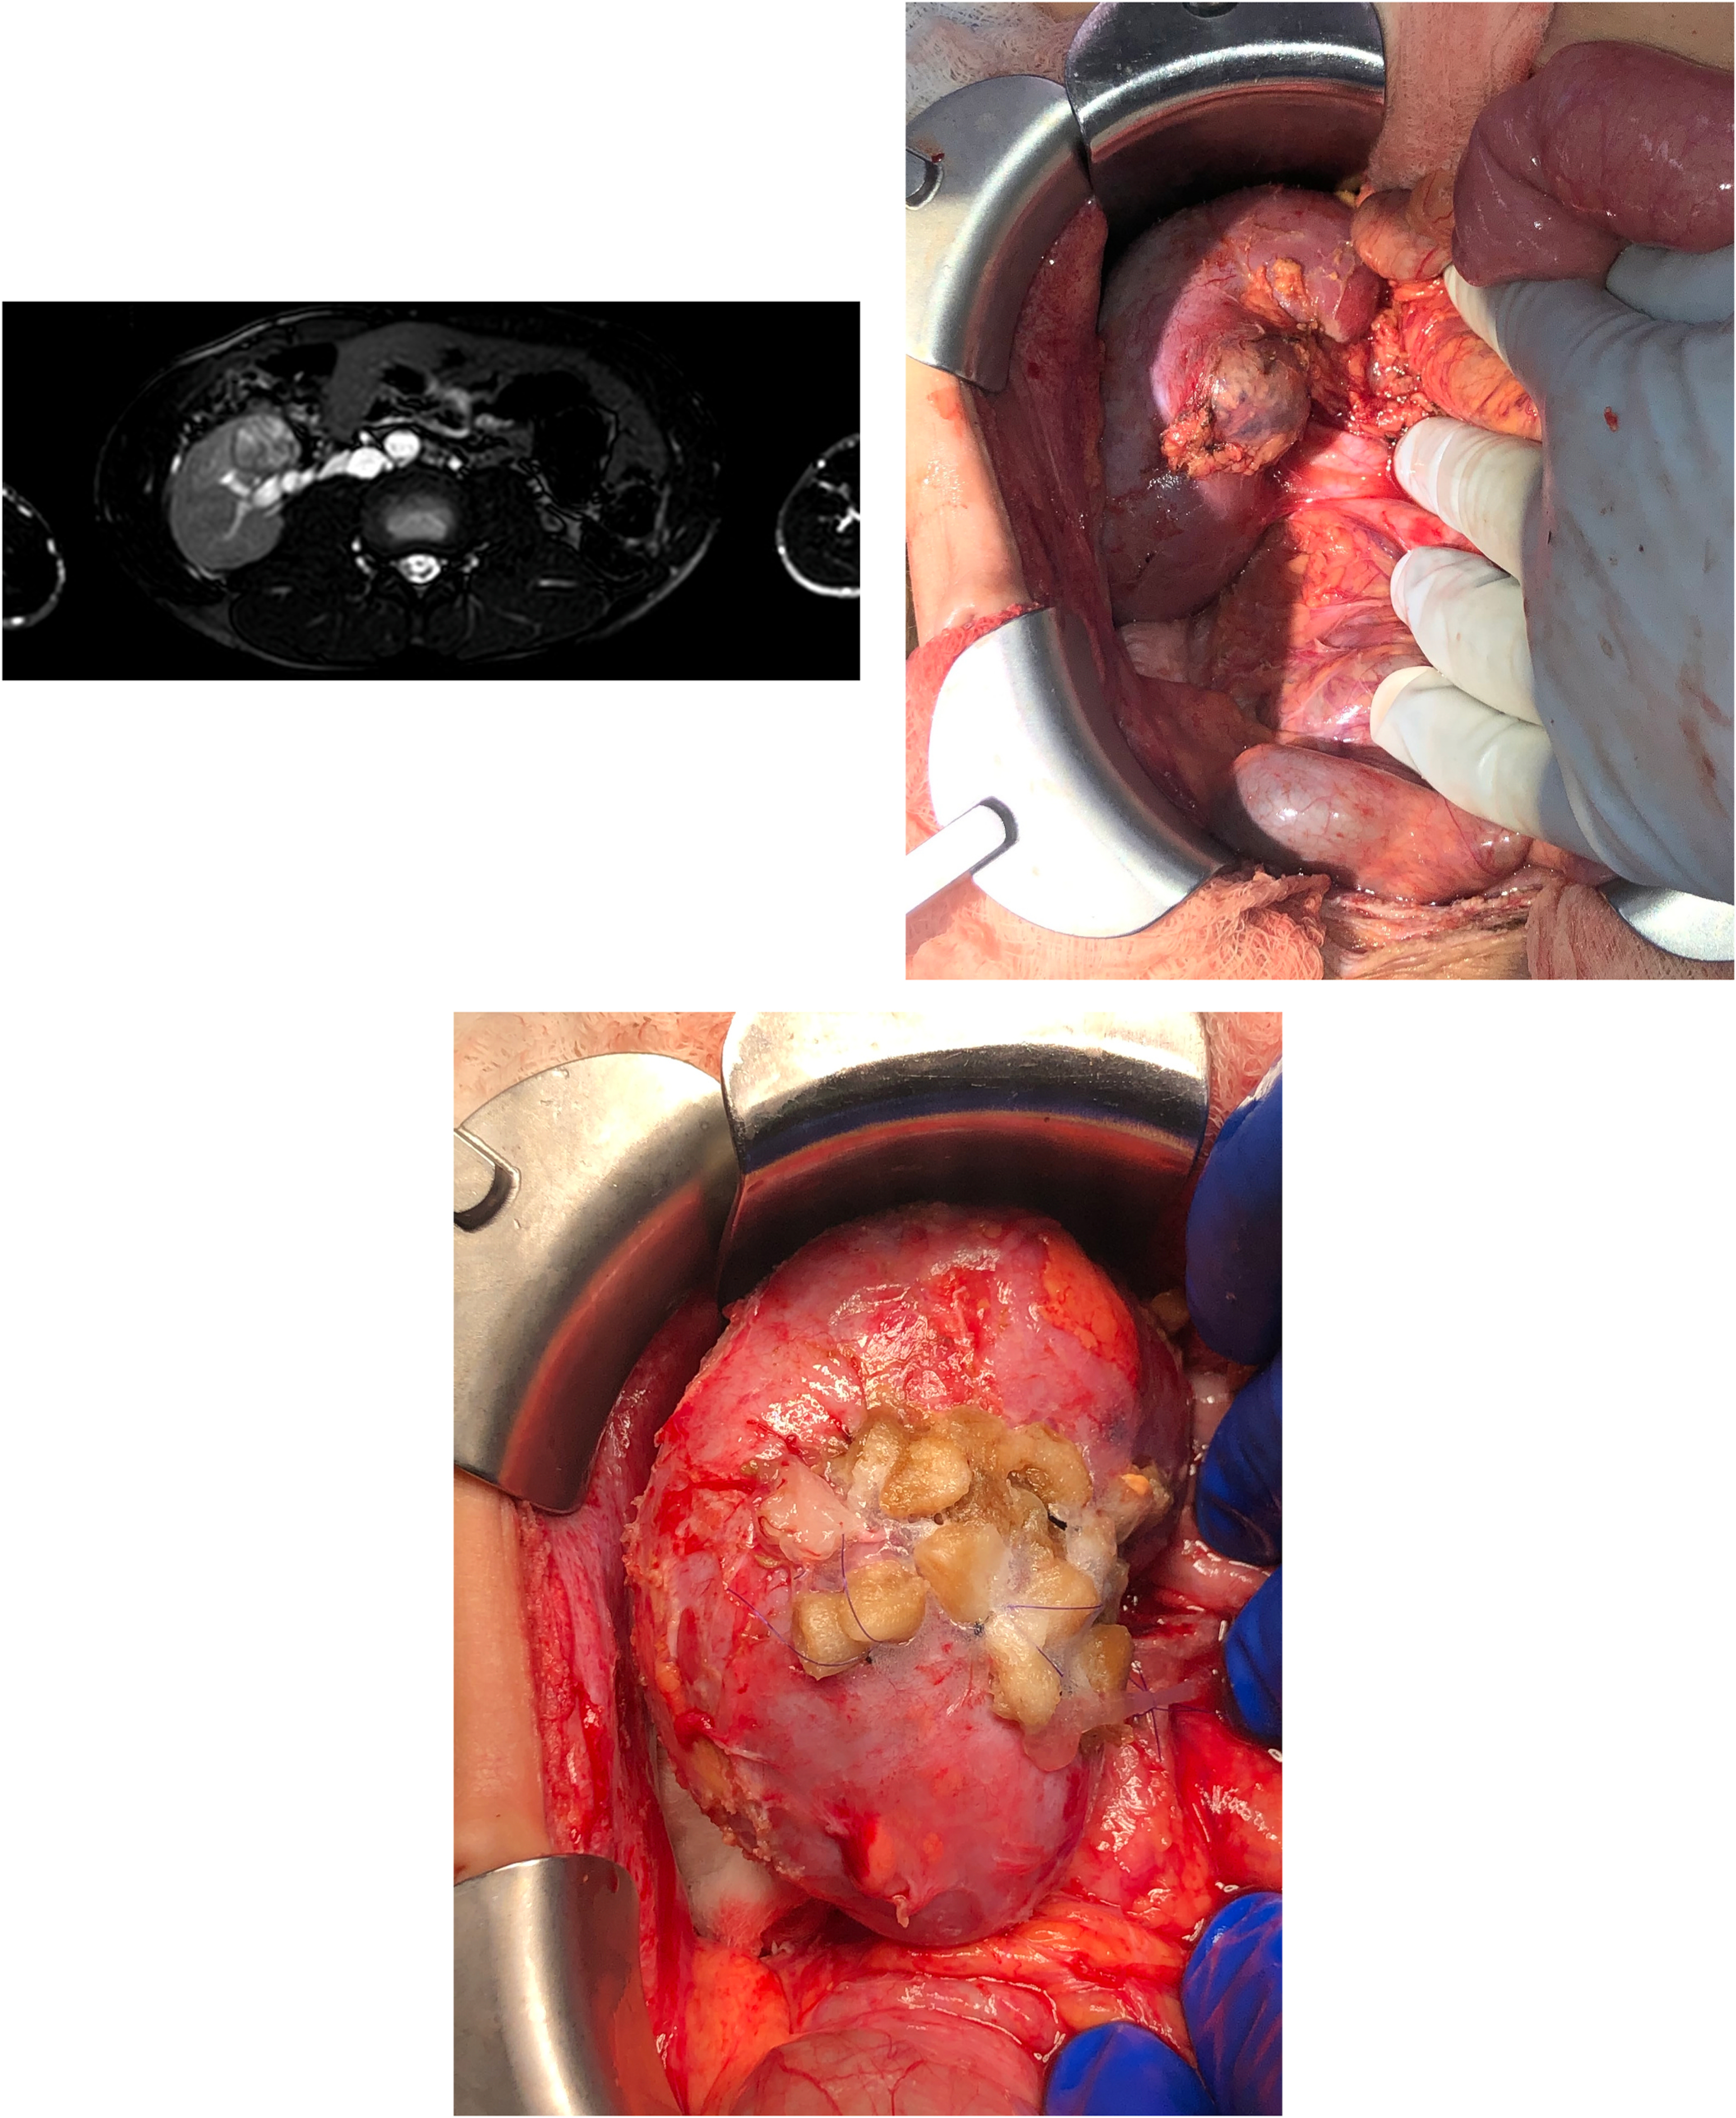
A, MRI of the abdomen showing the tumor in the allograft measuring 2.5 cm in diameter near the hilum and in close proximity to the collecting system; B, Intraoperative image showing the tumor close to the hilum and collecting system; C, Intraoperative image showing the kidney after performing partial nephrectomy. The collecting system was reconstructed, and the boundaries of normal renal parenchyma were oversewn. The edges of the open kidney were approximated with pledgets.
